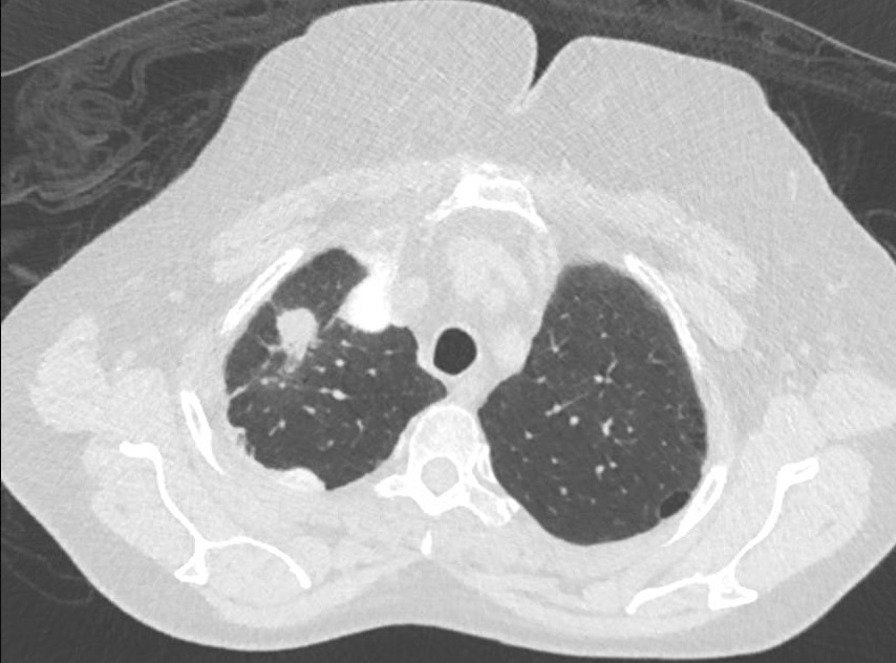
Computed tomography scan showing a right upper lobe lung lesion
